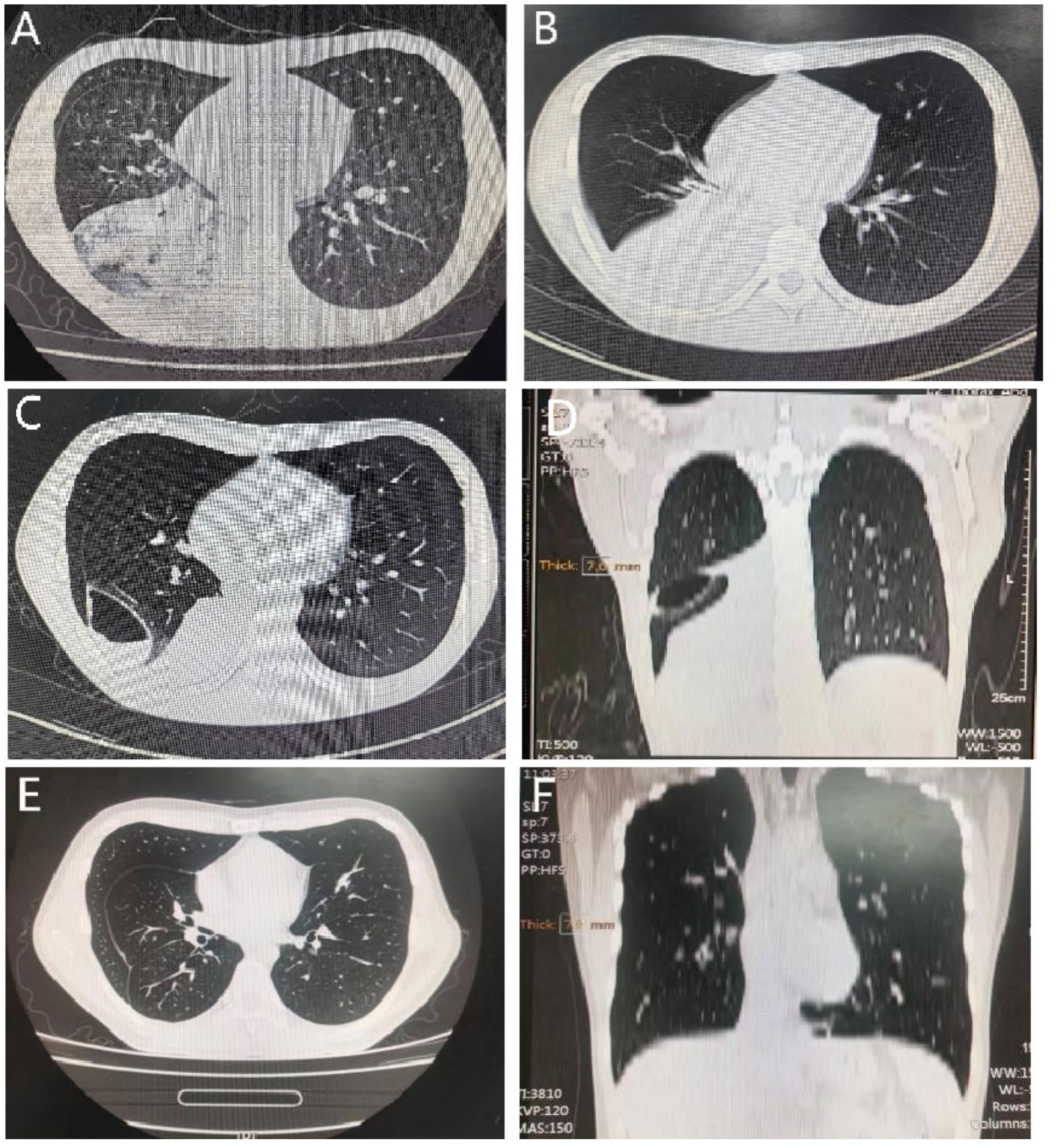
Chest CT images of the patient at different stages. Chest CT scans on May 21, 2022 (A), and May 25, 2022 (B). Chest CT on May 31, 2022 in transverse (C) and coronal views (D). Reexaminations of chest CT with the axial (E) and coronal views (F).
